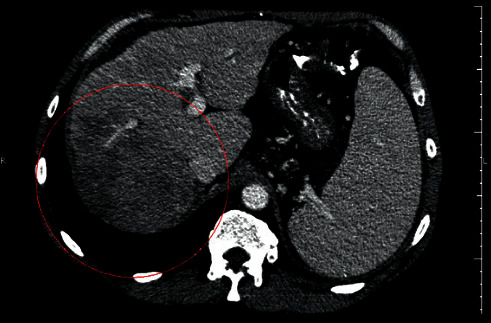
Computed tomography performed on February 18, 2019, showing a new progression of the tumor dimensions, now achieving 11.7 cm. The image was obtained during the arterial phase, but the arterial enhancement was changed to a radiolucent area underscored by the red circle.
